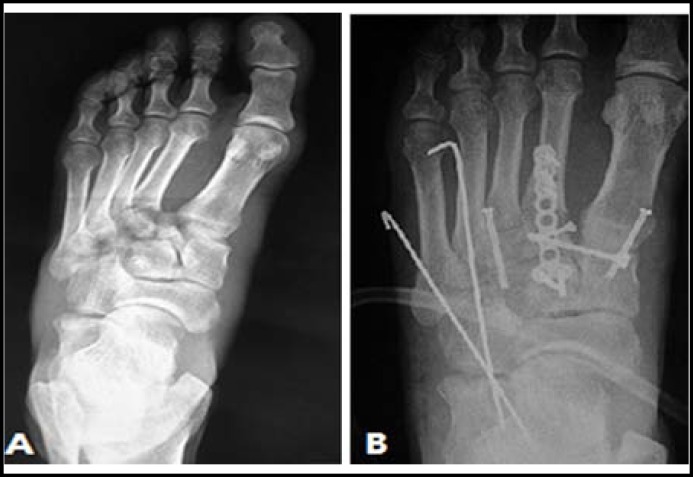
The use of K-wire to treat the injuries to the fourth and fifth TMT joints. A: Preoperative radiograph indicated Myerson type A injury with all the TMT joints dislocated laterally. B: Postoperative radiograph indicated the anatomic reduction with the fourth and fifth TMT joints being fixated with K-wires
